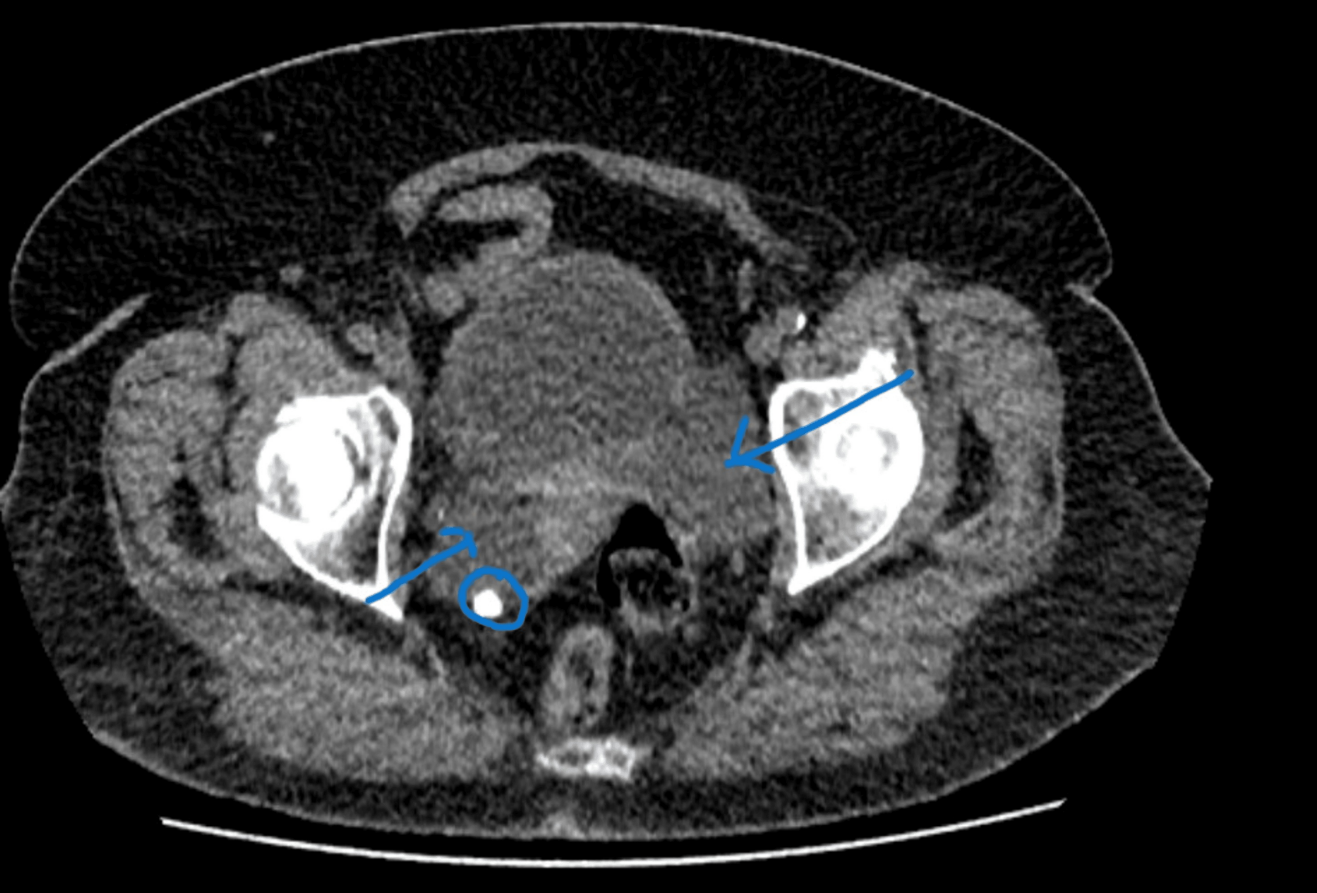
CT axial imaging showing bilateral dilated distal ureters (blue arrows) The right ureter contains the radiopaque stone.
